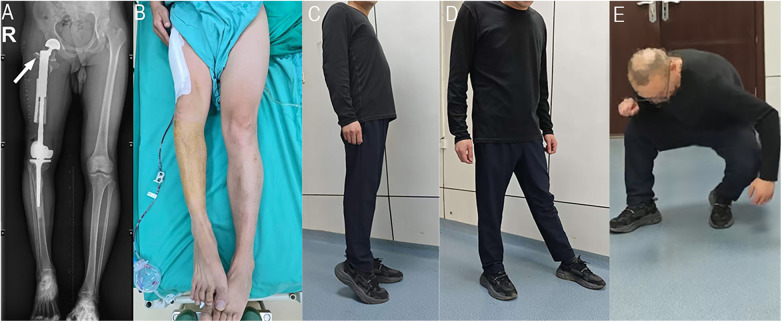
Postoperative outcomes. (A) Full-length anteroposterior radiograph showing satisfactory position of the customized construct; the arrow indicates the titanium-cable–reconstructed soft-tissue attachment site. (B) Supine photograph of the lower limbs. (C) Standing with the contralateral limb as the primary weight-bearing limb. (D) Standing with the affected limb as the primary weight-bearing limb. (E) Squatting posture.
